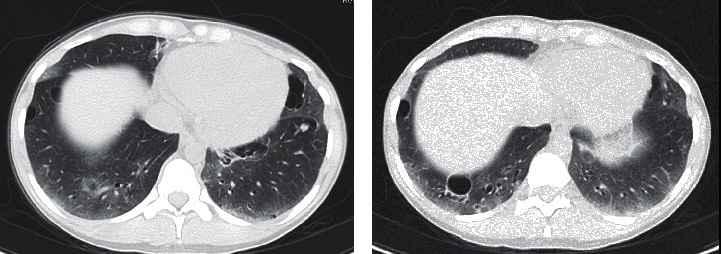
High-resolution computed tomography of the chest (Case 3). The scan prior to our evaluation (a) demonstrated pulmonary nodules, thin-walled cysts, basilar predominant ground glass attenuation, and subpleural consolidation consistent with lymphocytic interstitial pneumonia (LIP) in the setting of Sjögren's syndrome. A repeat scan (b) demonstrated increased ground glass attenuation and stable thick-walled cysts, and a few of the bilateral solid pulmonary nodules had slightly increased in size.
